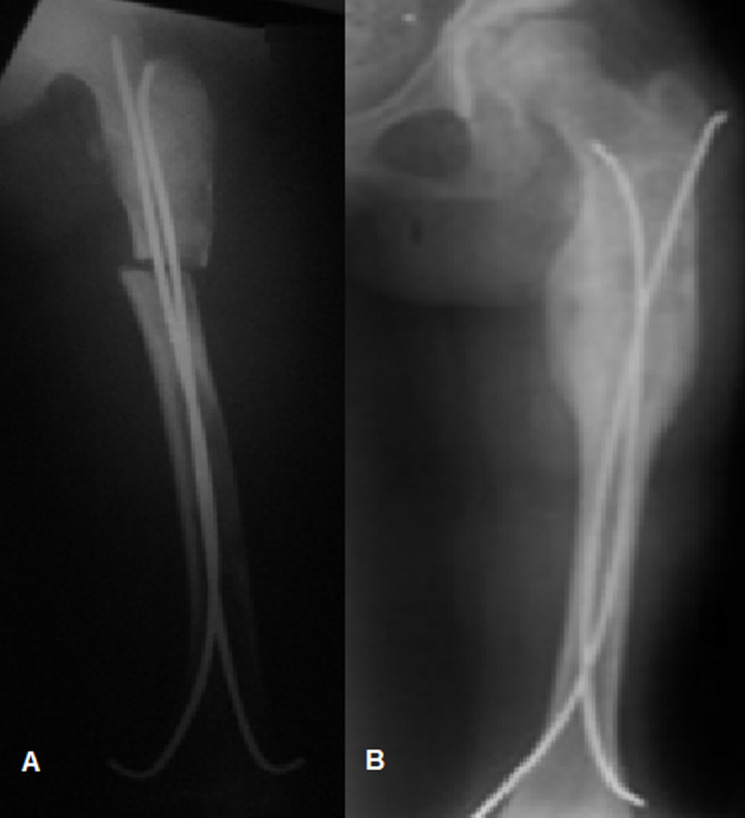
radiographie standard du fémur de face ; A) en post opératoire immédiat; B) à 3 mois post opératoire
